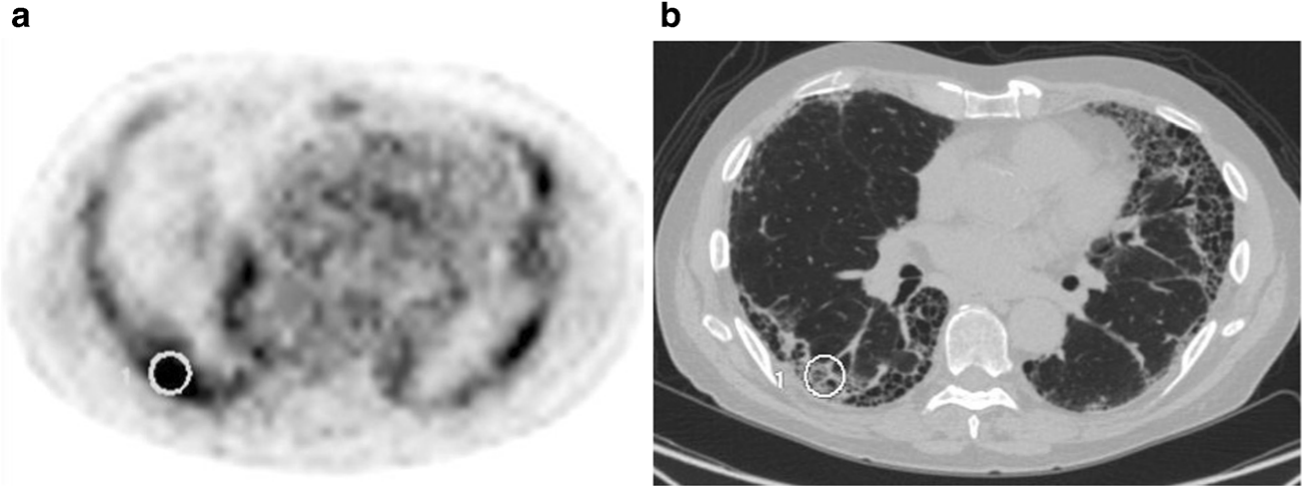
Co-registered PET (a) and CT (b) of a patient with IPF showing region of interest placement as part of measuring maximal pulmonary ^18^F-FDG uptake [7]. The *dark grey/black* regions are regions of high^18^F-FDG metabolism on the PET image (a)
